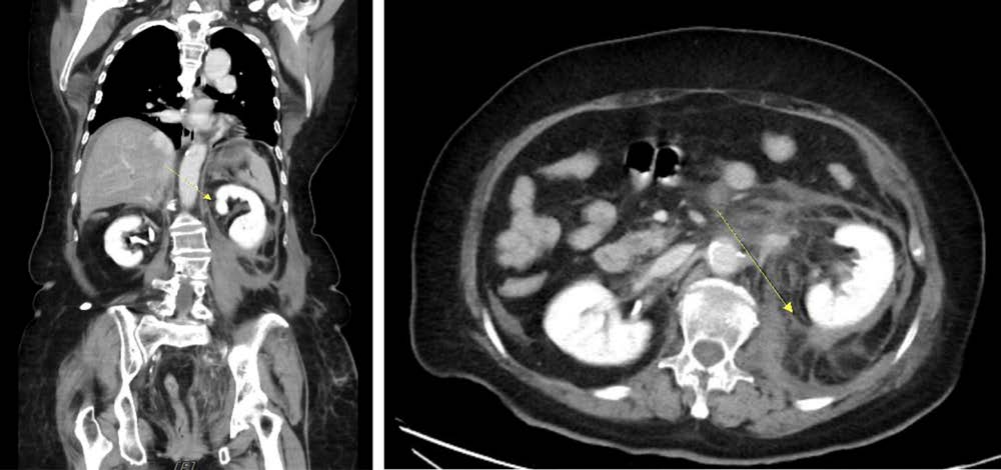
Abdominal CT scans revealed a left retroperitoneal hematoma, as indicated by the left arrow in both the axial and coronal views, respectively. CT = computed tomography.
